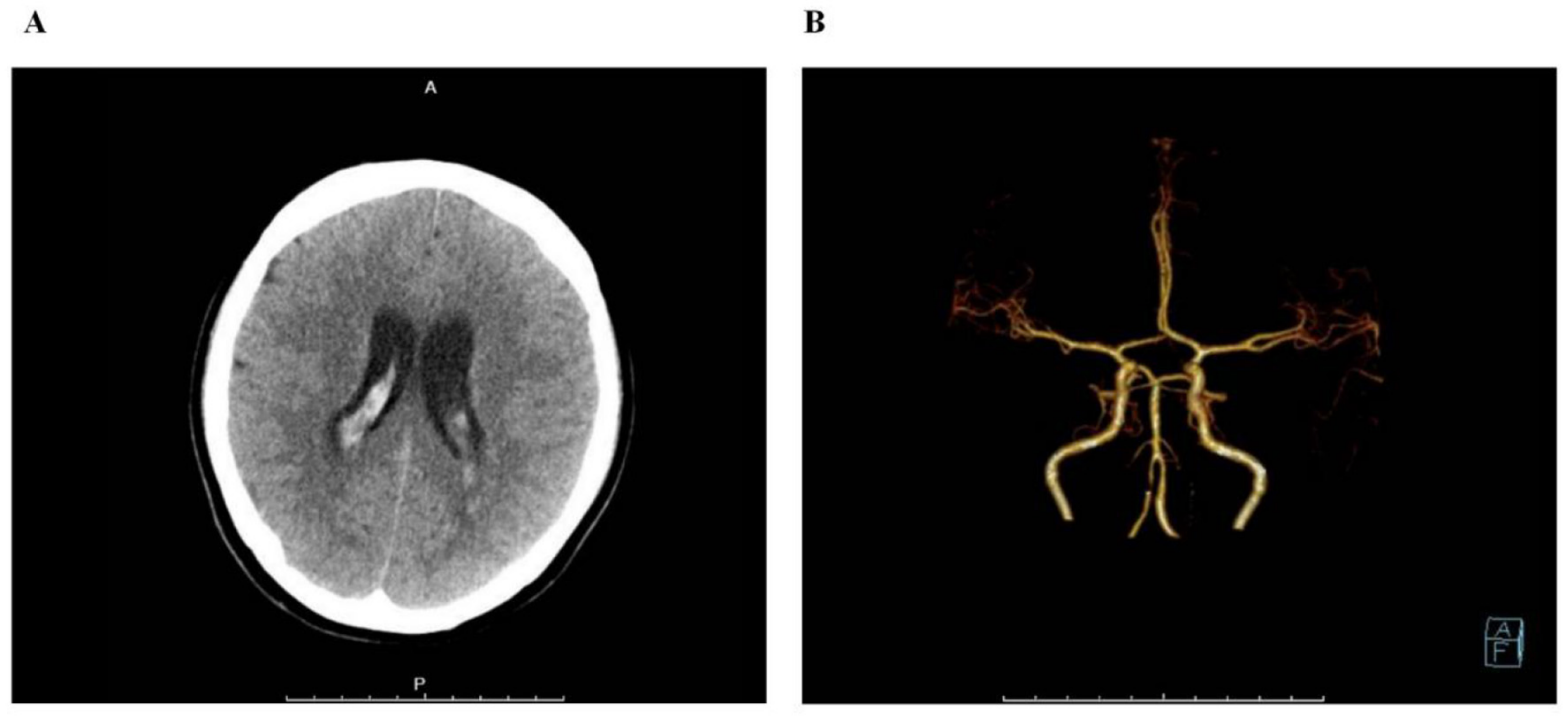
(A) Non-contrast CT scan on admission showing hyperdense blood filling the ventricular system. (B) CTA 3D reconstruction showing no visible vascular abnormalities.
